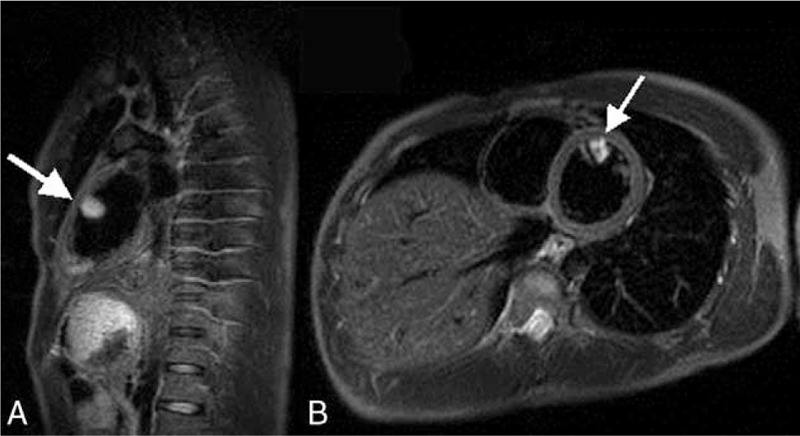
T1-weighted cardiac MR images showing that the mass (arrow) is attached to the anterior interventricular septum and anterior free wall of the left ventricle. MR = magnetic resonance.
